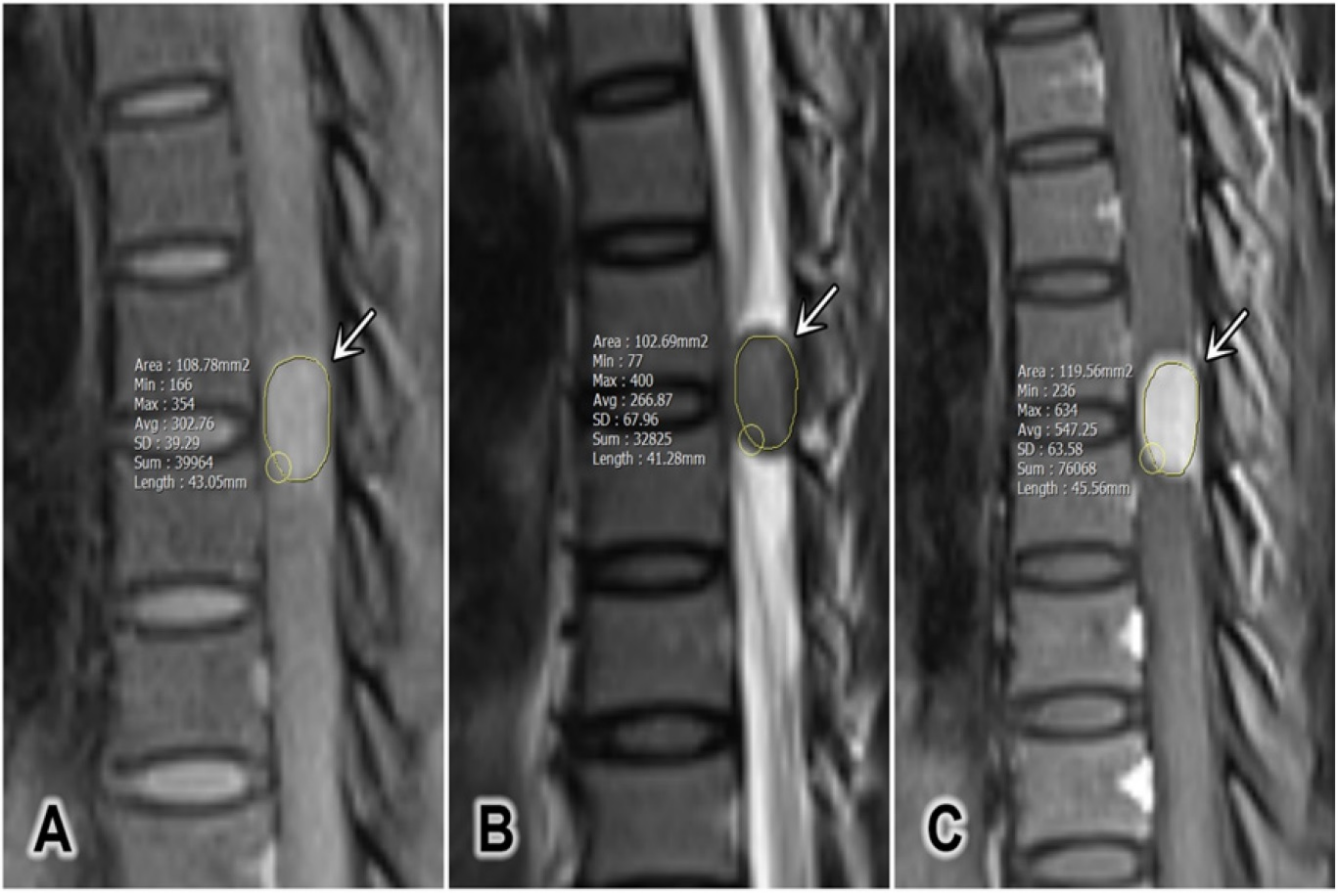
A representative region of interest defined for a tumor (white arrows). (A) Sagittal T1-weighted image, (B) sagittal T2-weighted image, and (C) sagittal contrast-enhanced T1-weighted fat-saturated image.
